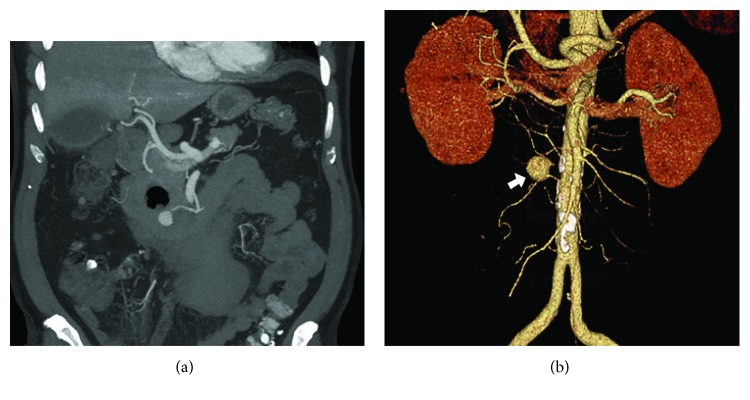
(a) Follow-up CT-scan: improved right upper quadrant inflammation, duodenal diverticulum (arrow), expanding pseudoaneurysm (arrow). (b) Angiography reconstruction: large pseudoaneurysm (arrow) of the pancreaticoduodenal artery.
